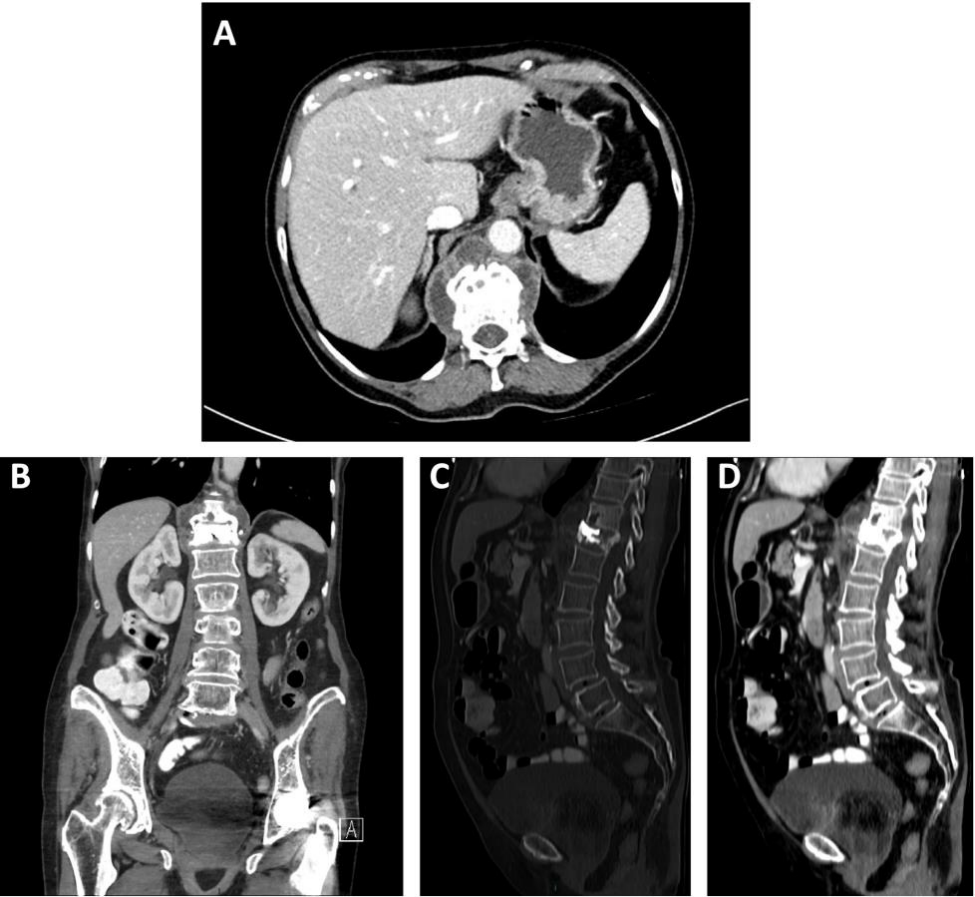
Contrast-enhanced Abdominal Images Computed Tomography Images. A: Axial soft tissue window at the level of D11–D12. B: Coronal soft tissue window. C: Sagittal bone window. D: Sagittal soft tissue window.
